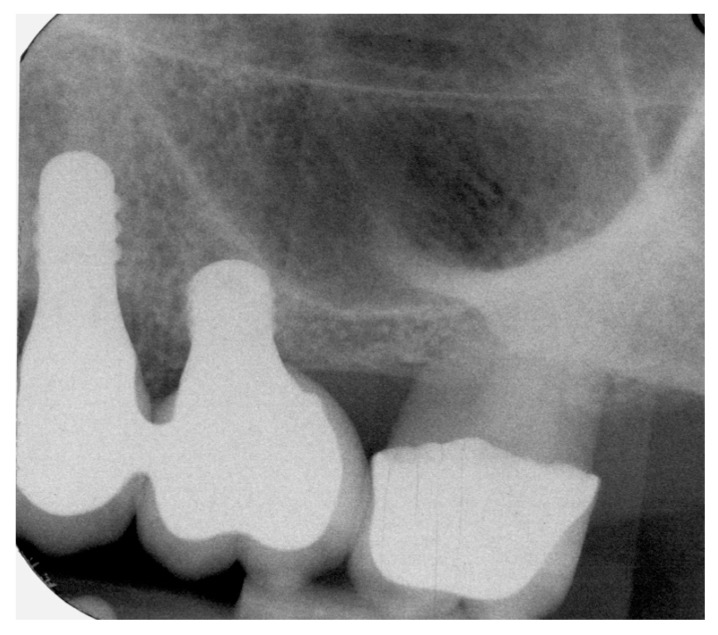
Radiograph of extra short and long implants at 12-month follow-up.
